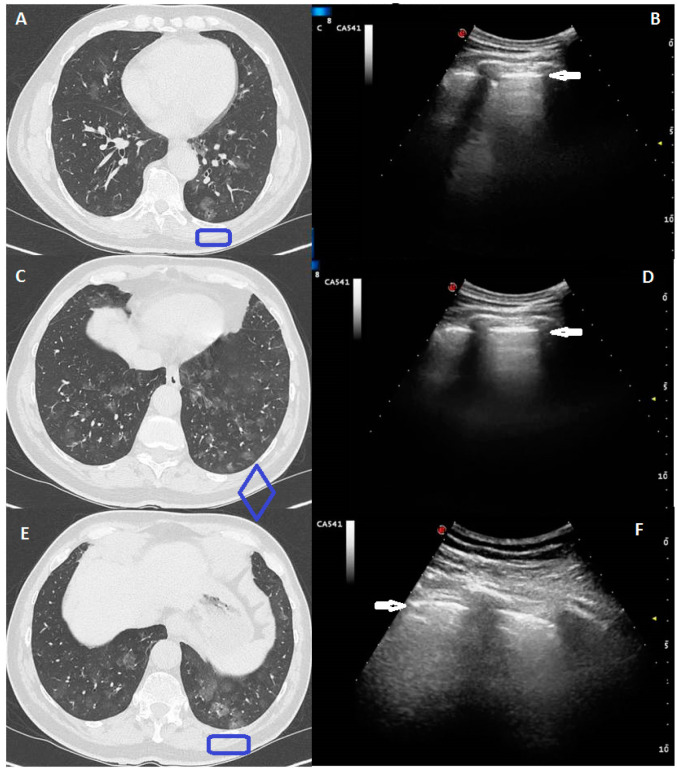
A 41-year-old male, presented with a one week history of persistent and worsening dry cough and fever with fatigue. The RT-PCR assay for the SARS-COV-2 showed a positive result. Computed Tomography (CT) scans in (A,C,E) show a diffuse pure bilateral ground glass opacity (GGO), also peripherally distributed, but not adherent to pleural surface. Ultrasound scans in (B,D,F) (corresponding to the blue boxes in the respective (A,C,E) CT scans), with a convex probe (6 MHz) and thoracic setting, do not show any pathologic pattern. The hyperechoic pleural line is highlighted by a white arrow.
